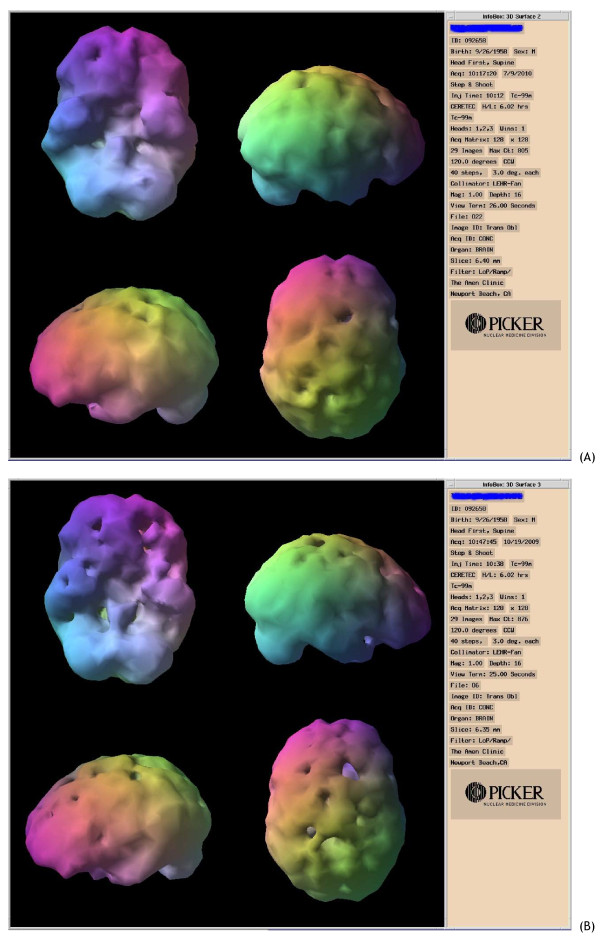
Pre and post HBOT SPECT imaging showing overall improved blood flow in the post HBOT scan (per Daniel Amen, MD).
